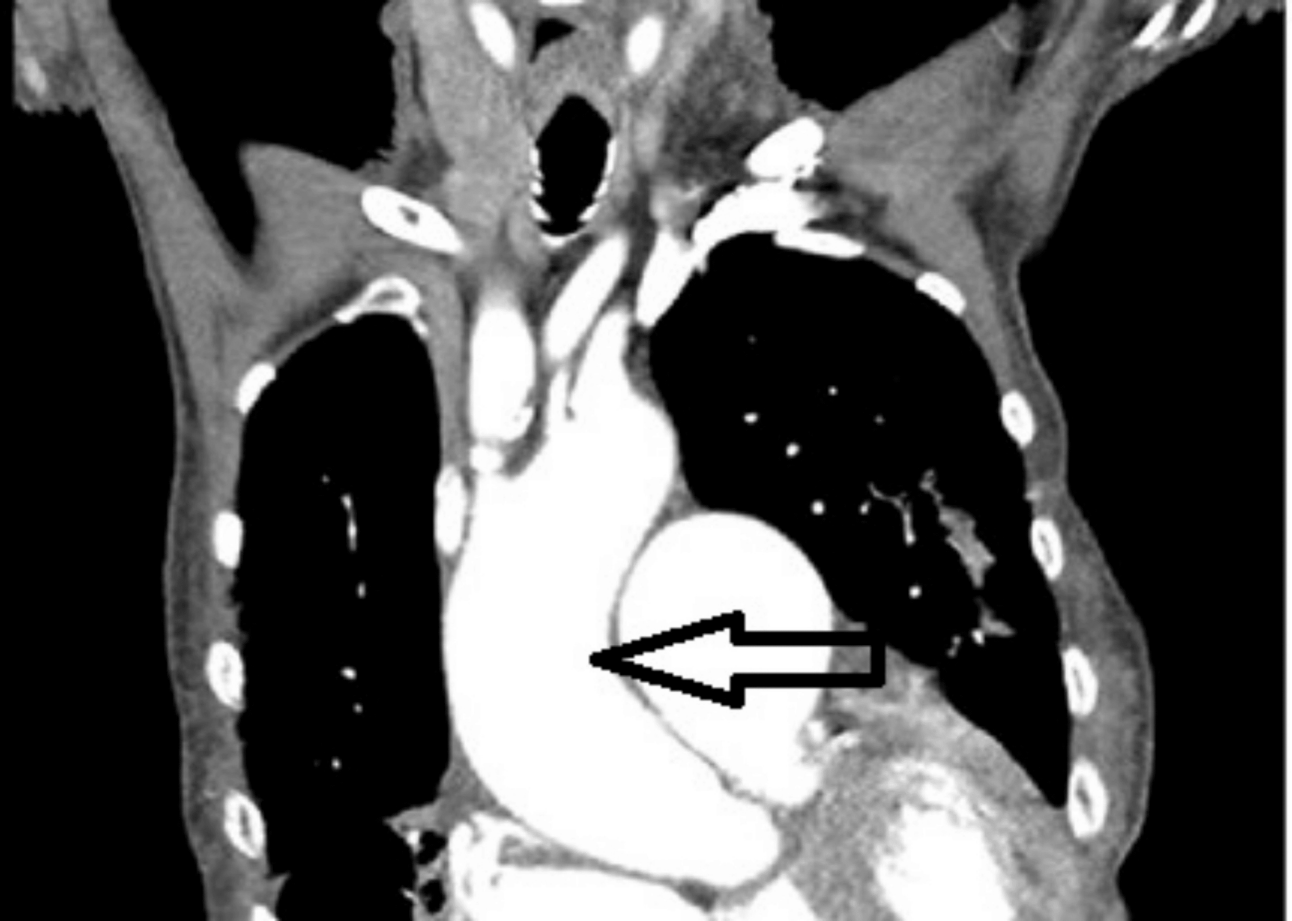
Dilated aortic root on a CT of the thorax (arrow) CT: computed tomography
